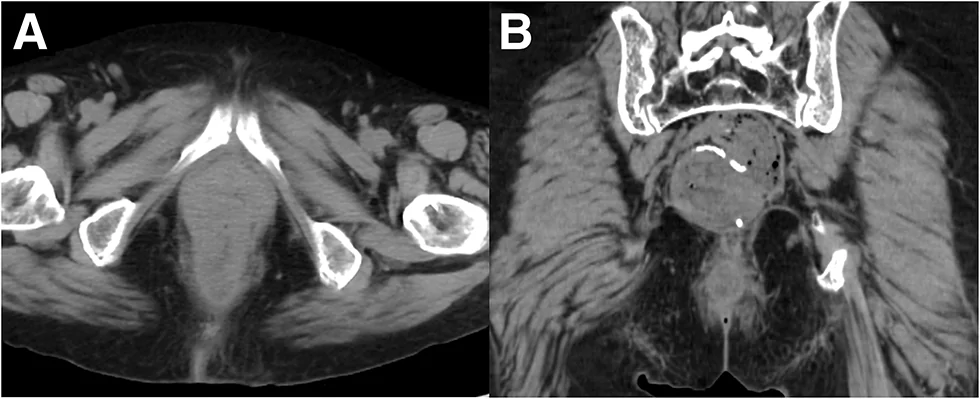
Pelvic CT imaging at the age of 73. (A) Axial view. (B) Coronal view. A 35 × 20-mm area with fluid collection was observed posterior to the rectal wall, accompanied by increased attenuation of the surrounding fat tissue, suggestive of a perirectal abscess.
